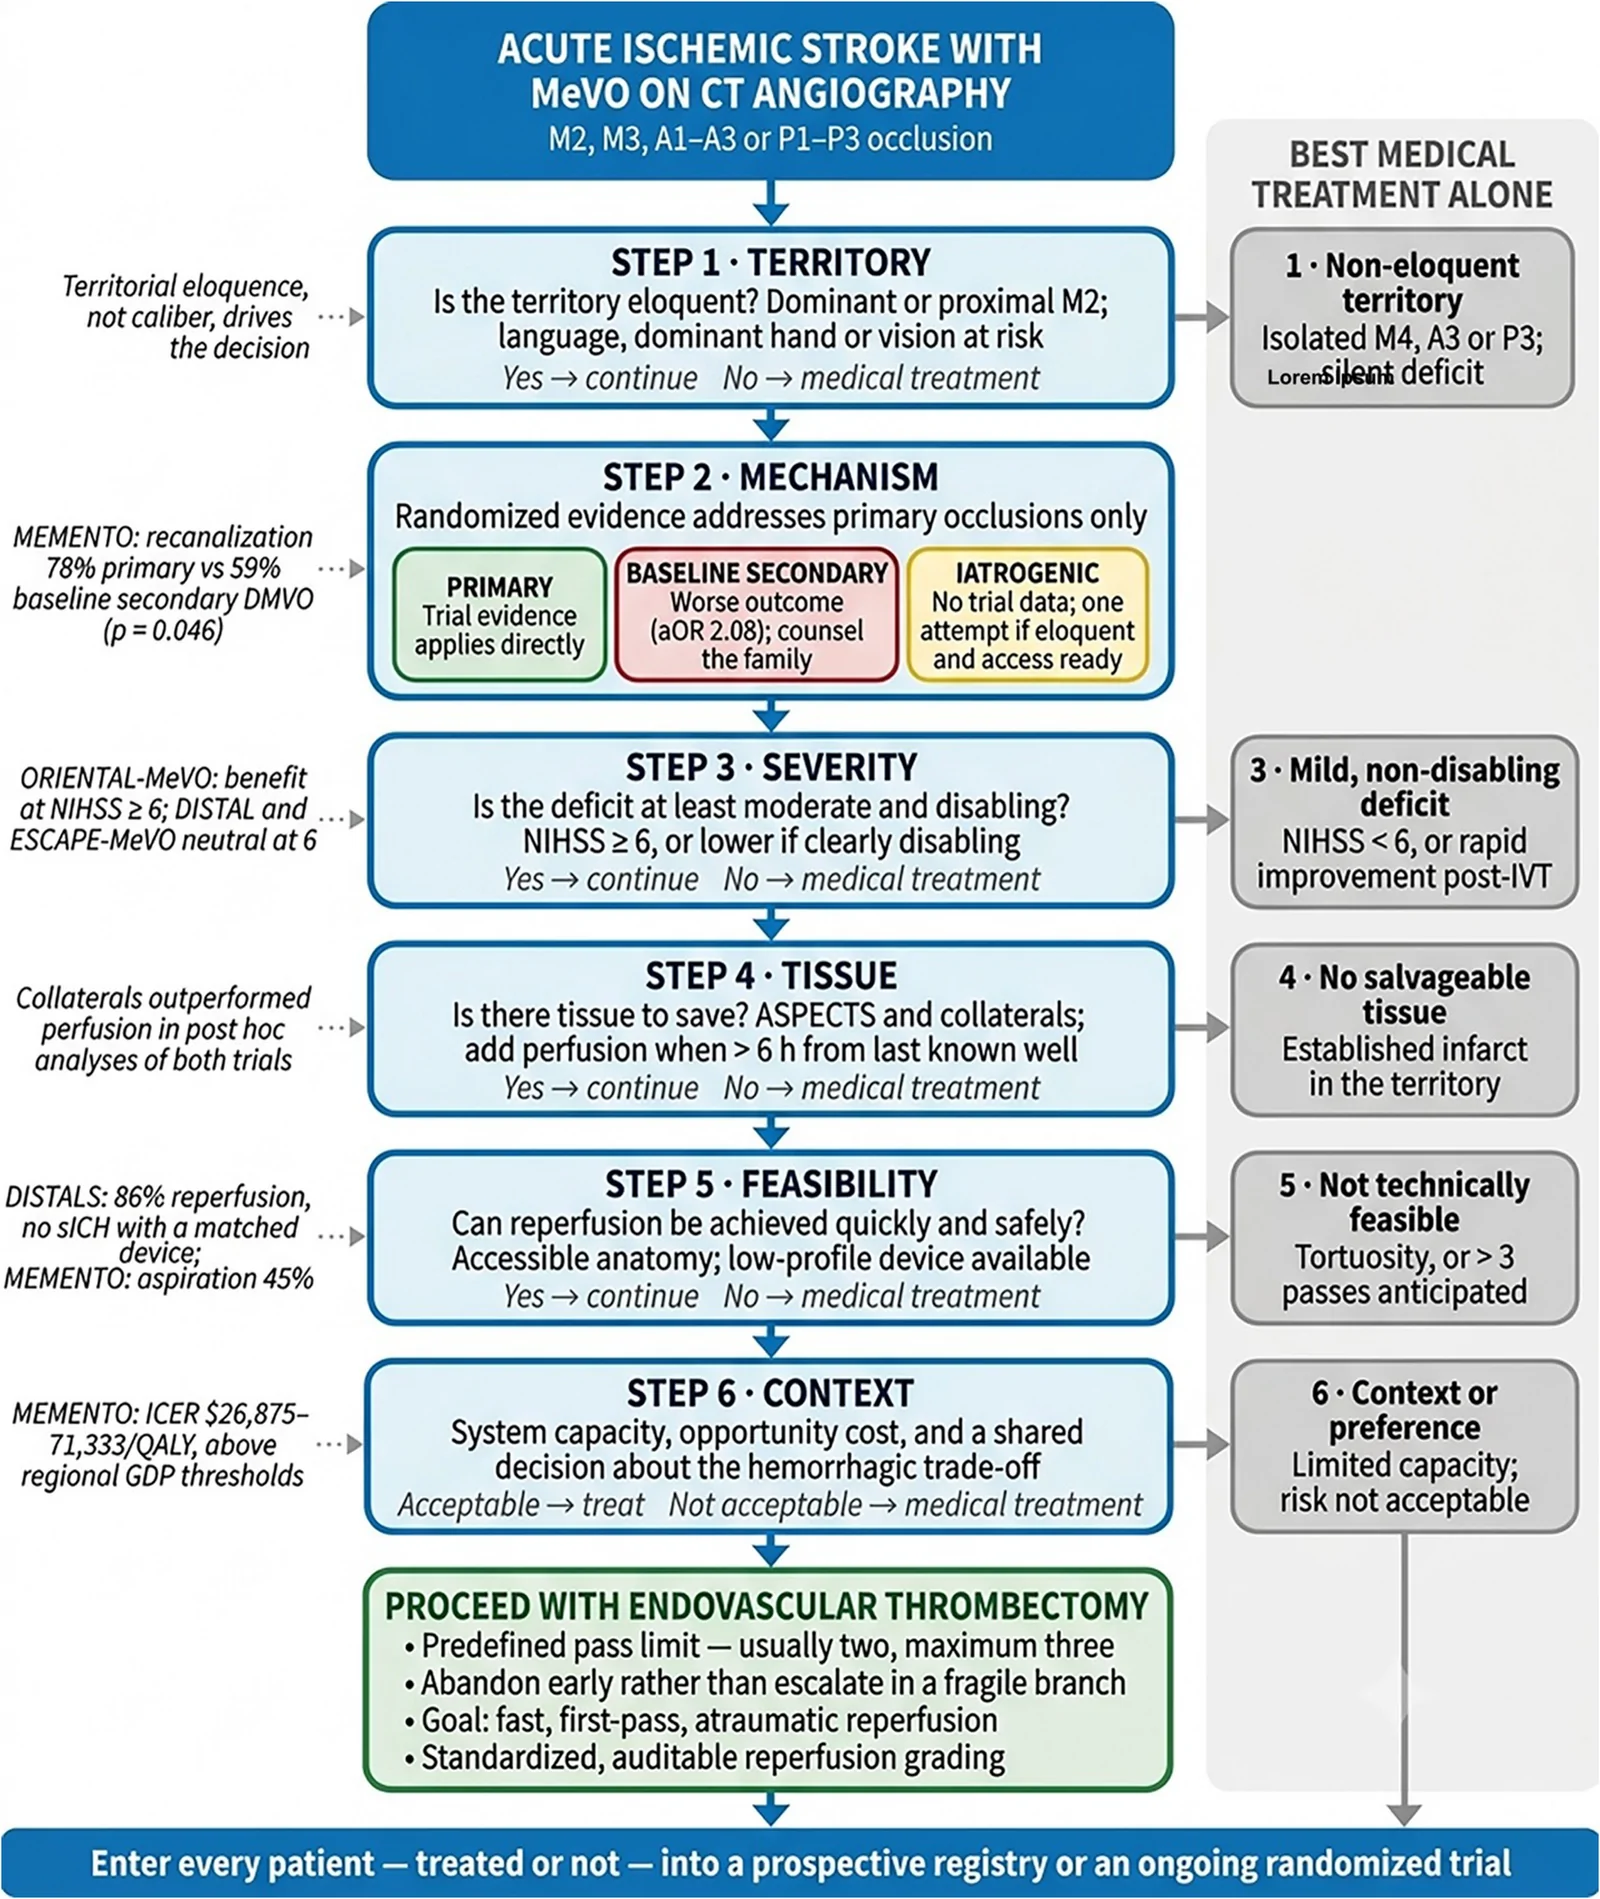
Proposed decision framework for acute medium vessel occlusion (MeVO) stroke in the post-trial era. Step 1: confirm MeVO on CT angiography and classify by territorial eloquence (dominant/proximal M2 and eloquent cortex versus non-eloquent M4/A3/P3). Step 2: determine occlusion mechanism — primary, baseline secondary (proximal clot fragmentation) or iatrogenic secondary (peri-procedural embolus) — since randomized evidence addresses primary occlusions only. Step 3: grade clinical severity (NIHSS ≥ 6 or clearly disabling deficit)
